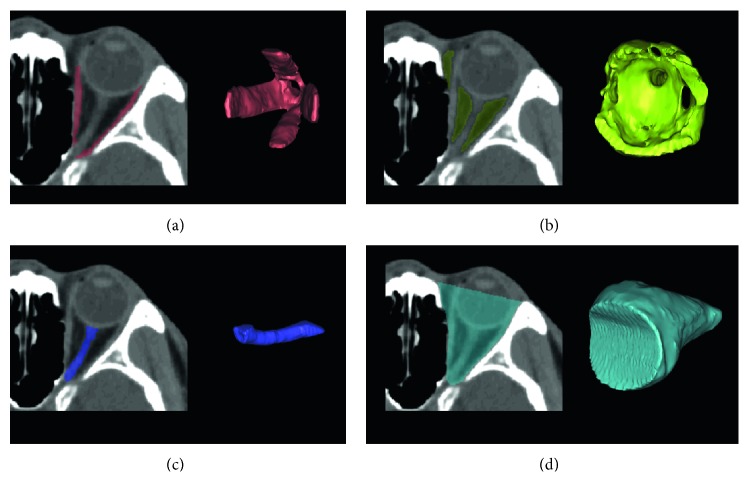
Axial CT slices with the highlighted segmented tissues and the three-dimensional reconstruction of the segmented issues: (a) extraocular muscle, (b) orbit fat, (c) intraorbital optic nerve, (d) orbital bony cavity.
